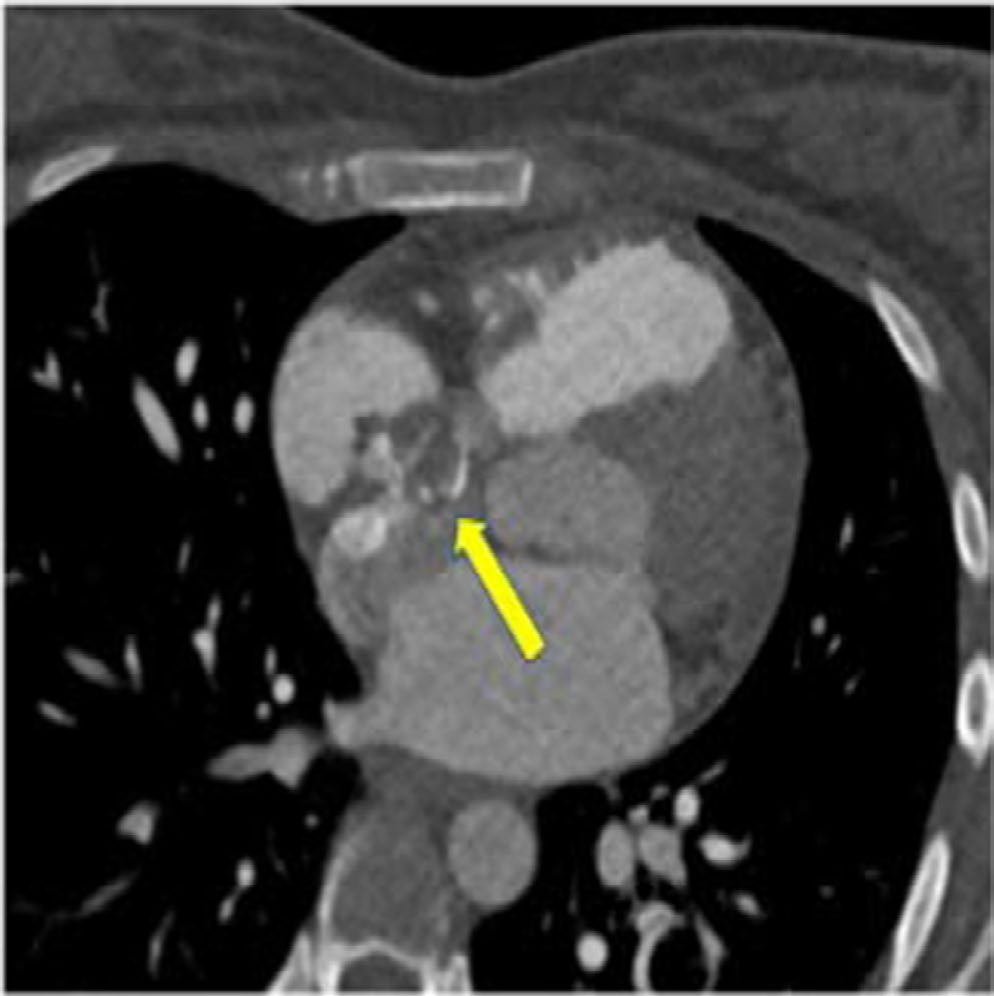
Axial CT pulmonary angiography: showing incidental right atrial filling defect (Yellow arrow)
